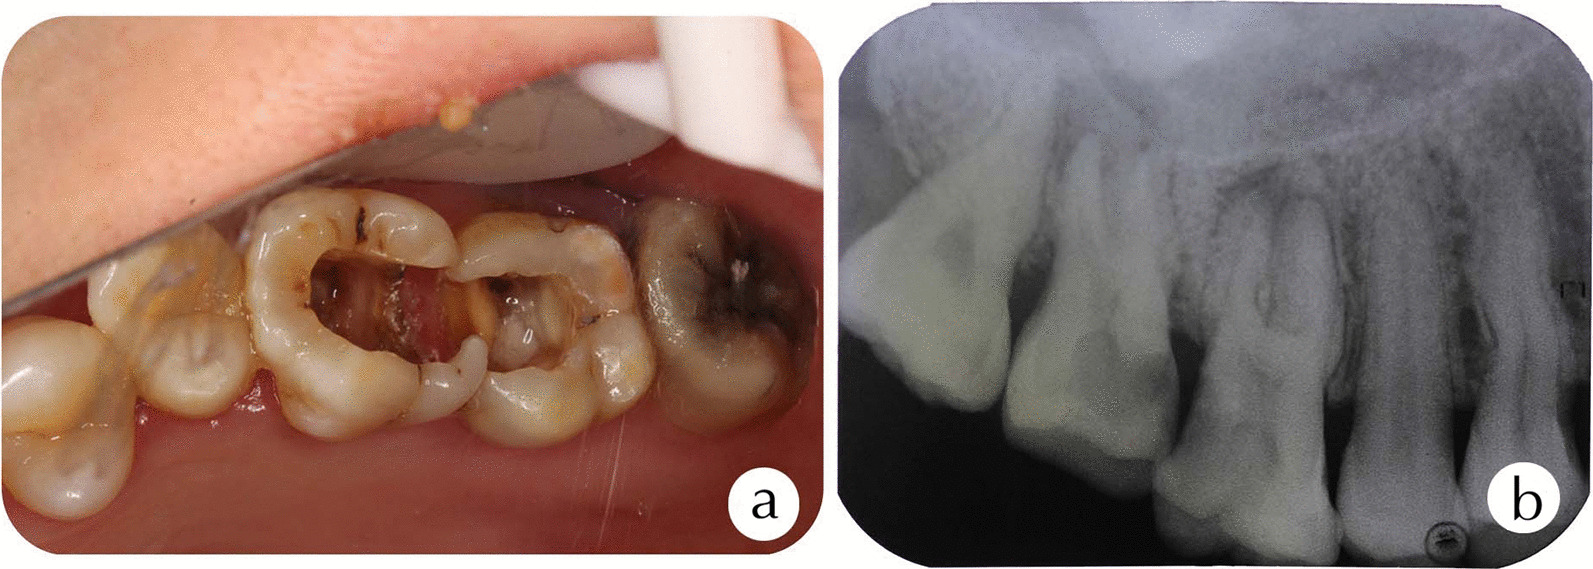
a The intraoral examination of teeth #16 and #17. b A preoperative radiograph of teeth #16 and #17
